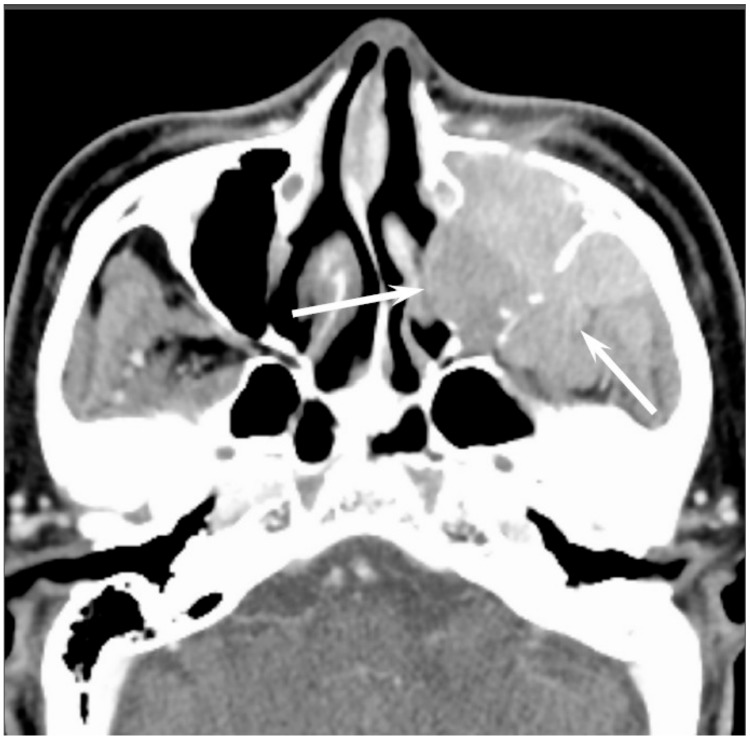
Diffuse large B-cell lymphoma of the left maxillary sinus. Contrast-enhanced CT image showing a homogeneously enhanced lesion accompanied by remaining sinus walls as a linear structure within the tumor (arrows).
